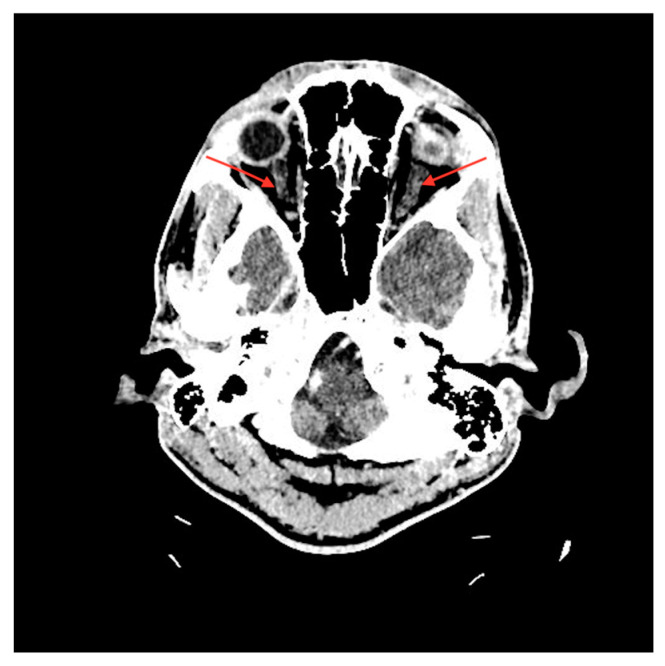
CT scan shows symmetrical thickening of optic nerve sheaths (red arrows).
